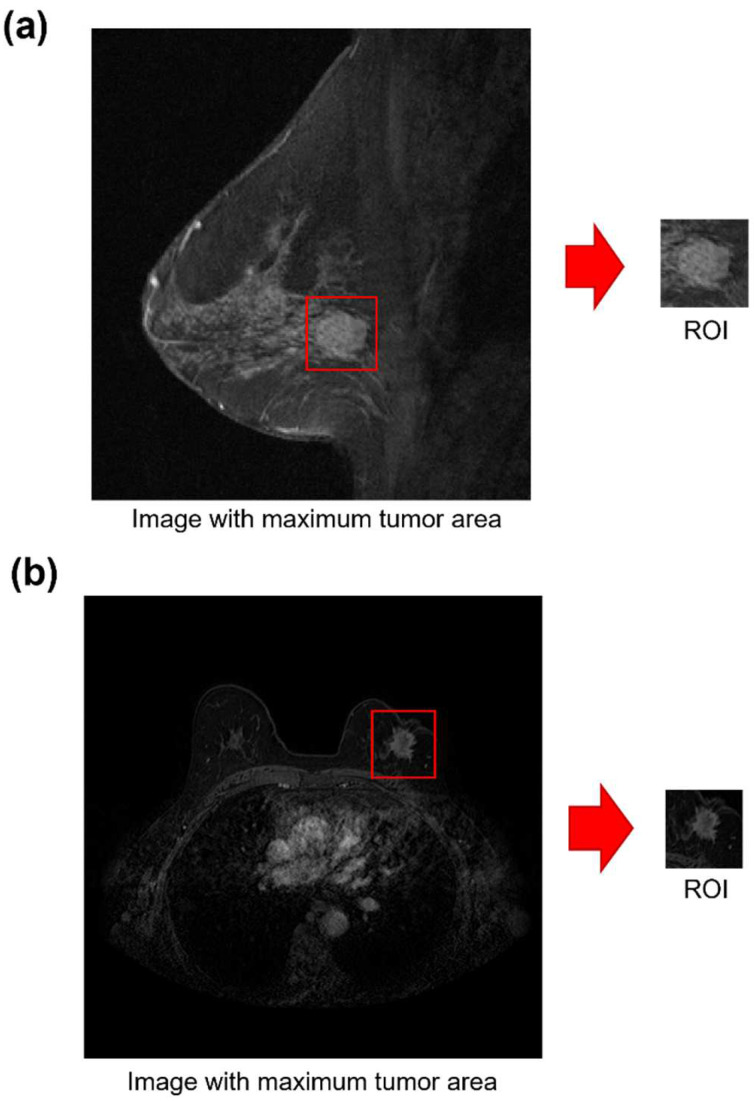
Examples of baseline MR image acquired according to (a) a sagittal view of a public DB patient and (b) an axial view of a private DB patient.
